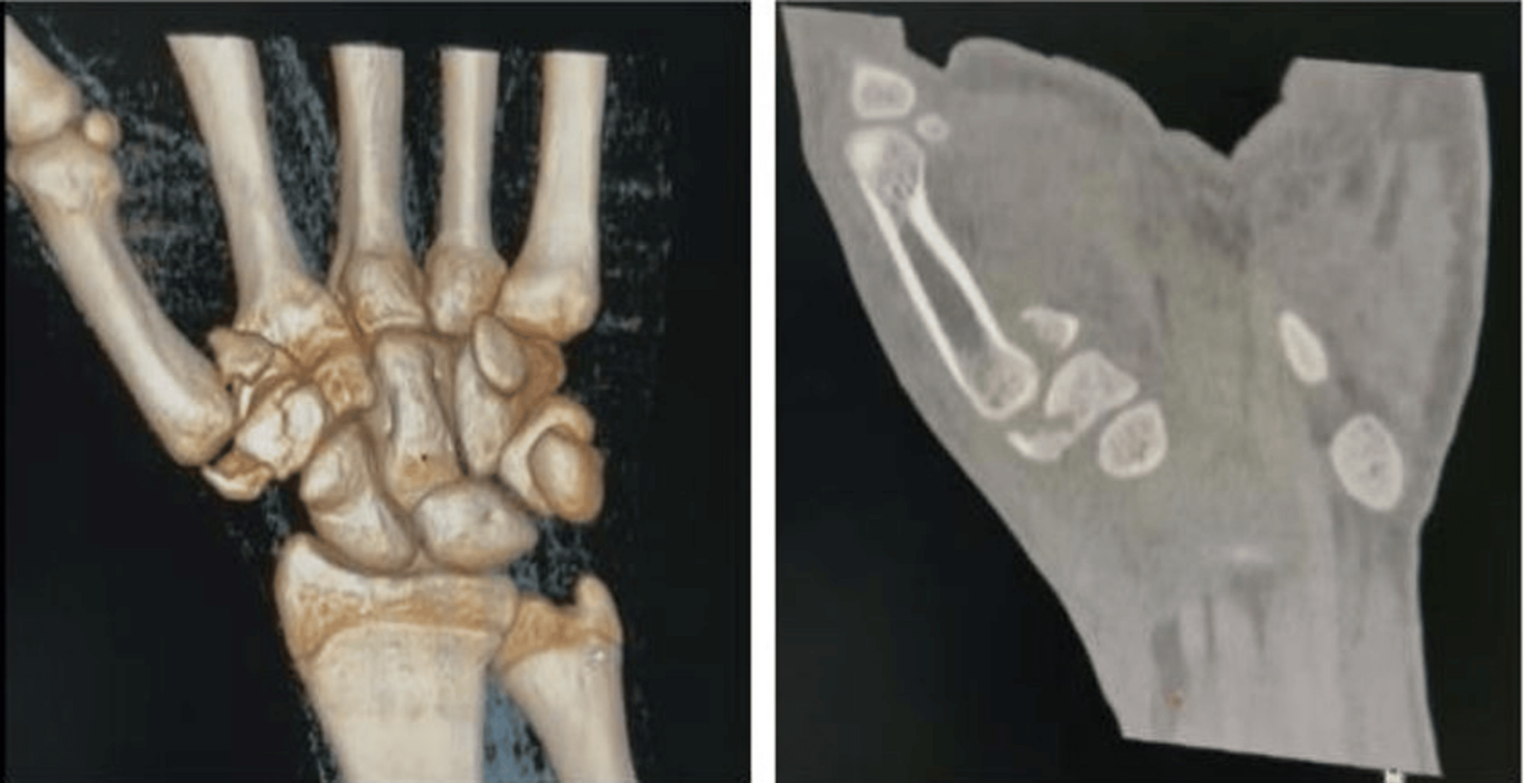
Computed tomography (coronal sections + 3D) of the left hand demonstrating a Bennett fracture associated with a vertical trapezium fracture.
